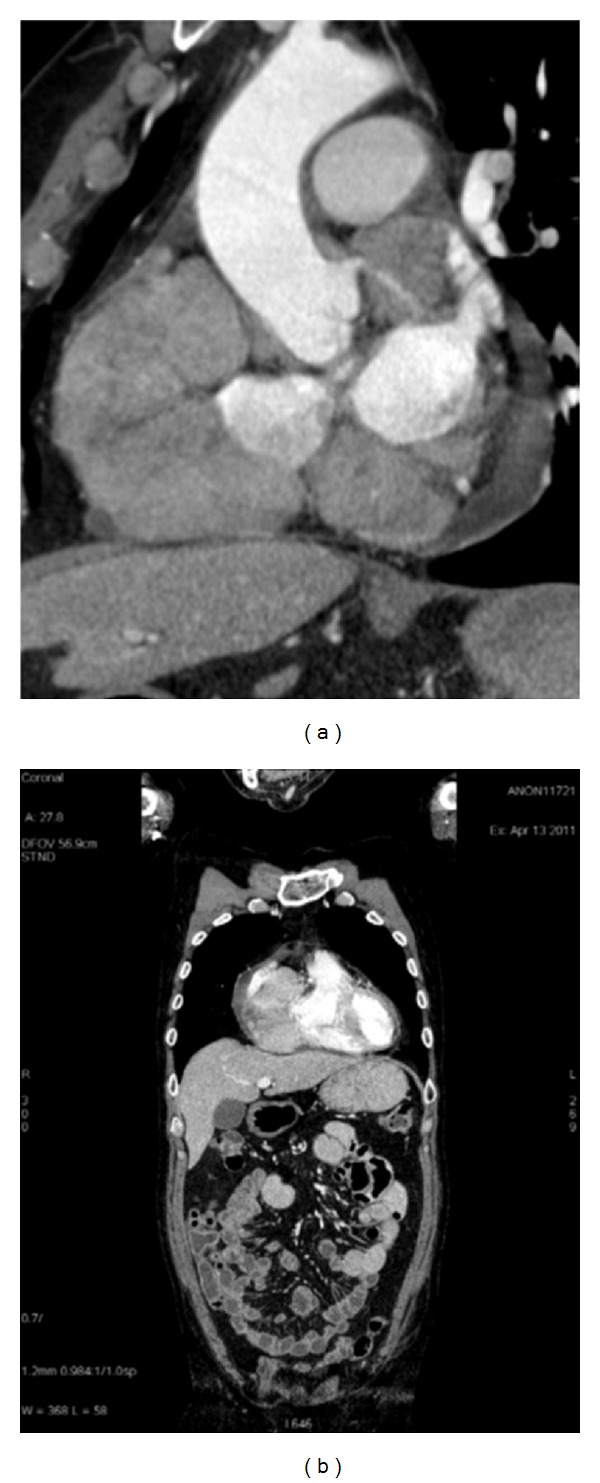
(a) Sagittal reconstruction; (b) coronal reconstruction. CT shows the presence of right atrium myocardial mass and no other abnormality in other organs.
